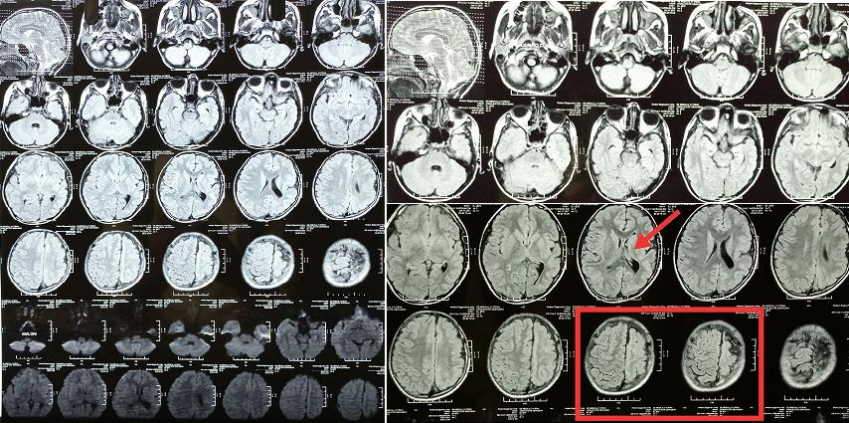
brain MRI findings shows left cerebral hemi-atrophy with thinning of the ipsilateral grey matter cortex, reduced volume of the underlying white matter (red box), enlargement of the left lateral ventricle (red arrow)
